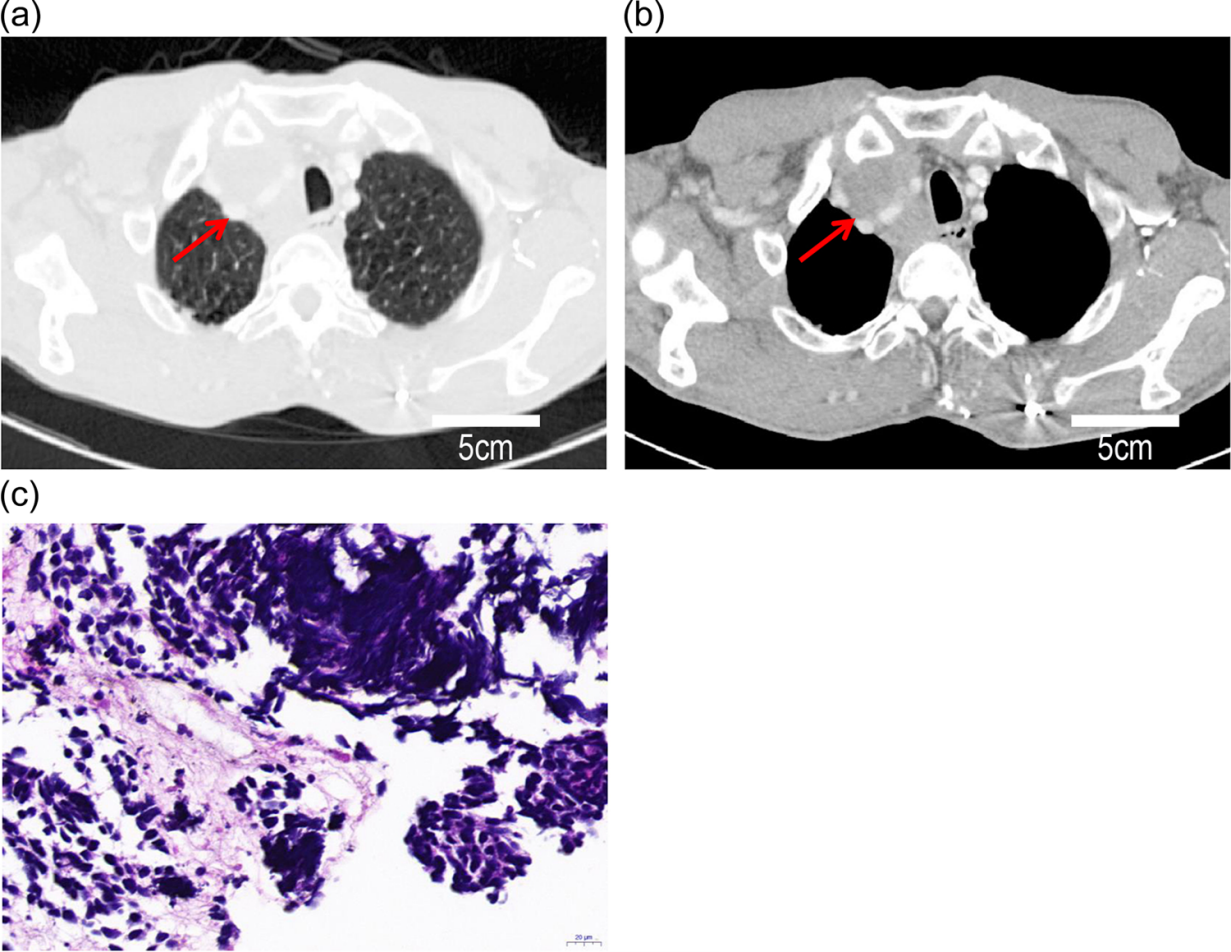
Observation of small cell cancer: (a and b) enhanced chest CT scan findings of a right upper mediastinal mass, contrast medium was iohexol and (c) pathologic findings of a right upper mediastinal mass (small cell cancer).
